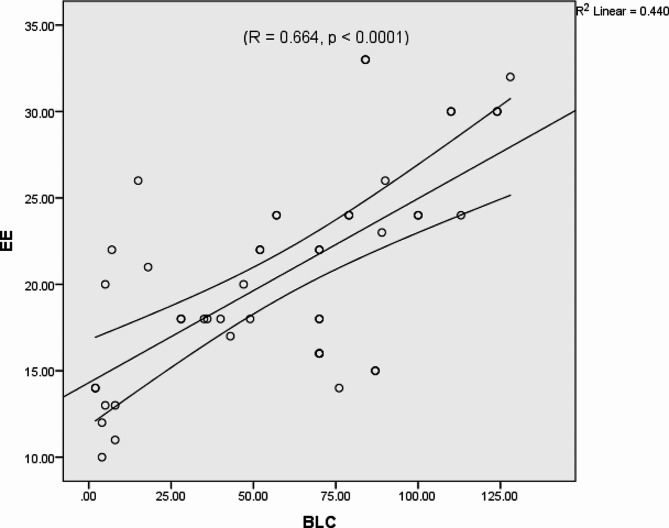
Correlation between B-line counts (BLCs) and tissue Doppler (EE) Doppler signals The standard R function for calculating Pearson correlation
